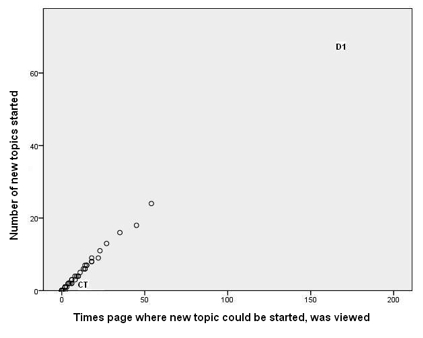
Number of topics started by each participant versus number of times they viewed the page from where new topics could be started. This shows two outliers: Discussant 1 (D1), who started many topics, and Caretaker (CT)
